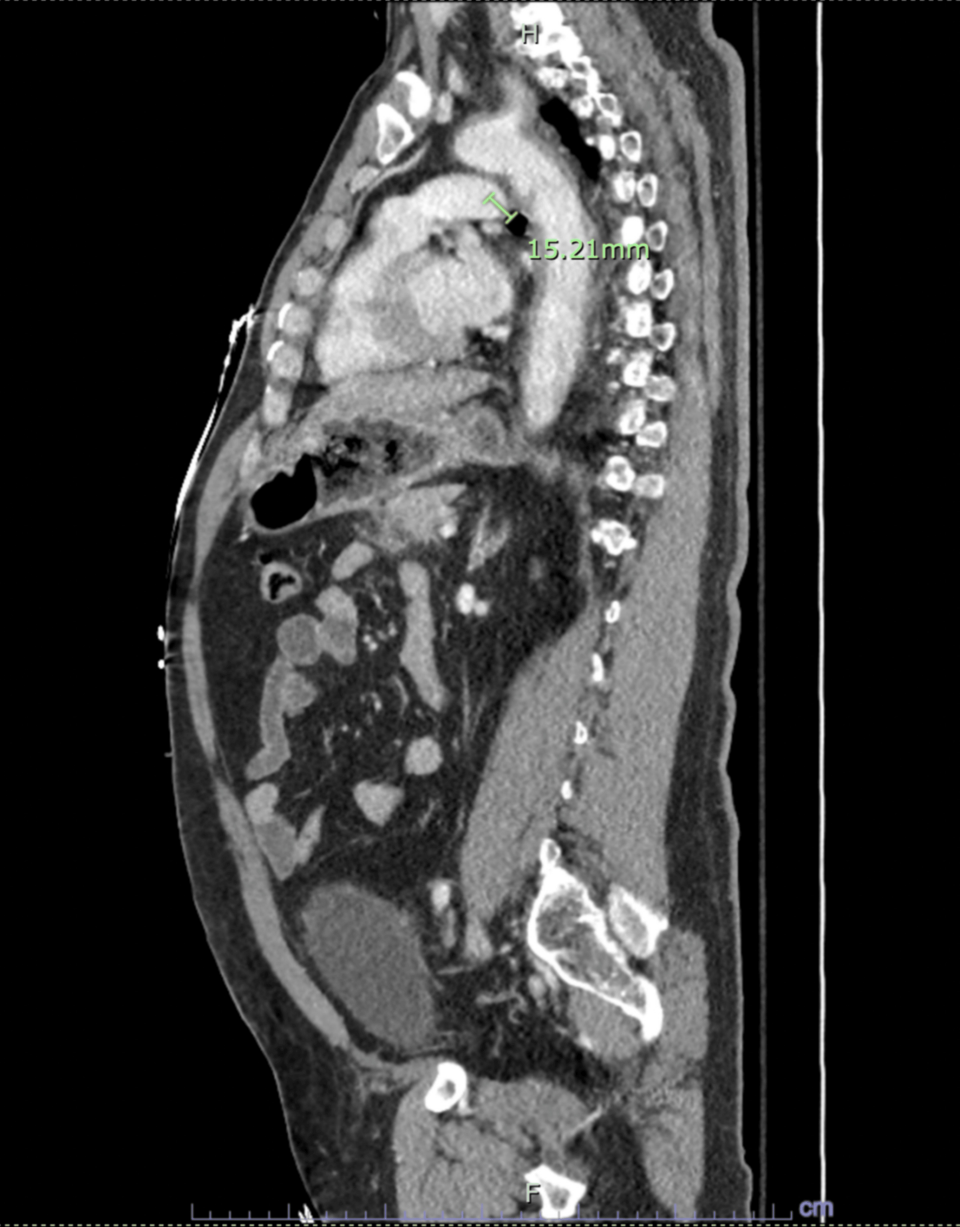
CT scan revealing a flap coursing across the medial wall of the descending aorta with an associated pseudoaneurysm measuring 1.5 cm protruding anteromedially.
